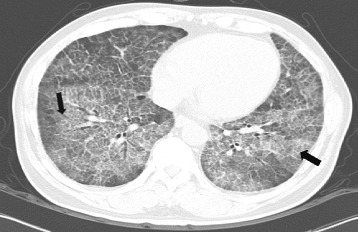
Contrast enhanced computed tomography revealing Crazy paving patterned diffuse ground glass attenuation with inter/intralobular septal thickening, representing diffuse alveolar damage, both lungs (black arrow).
